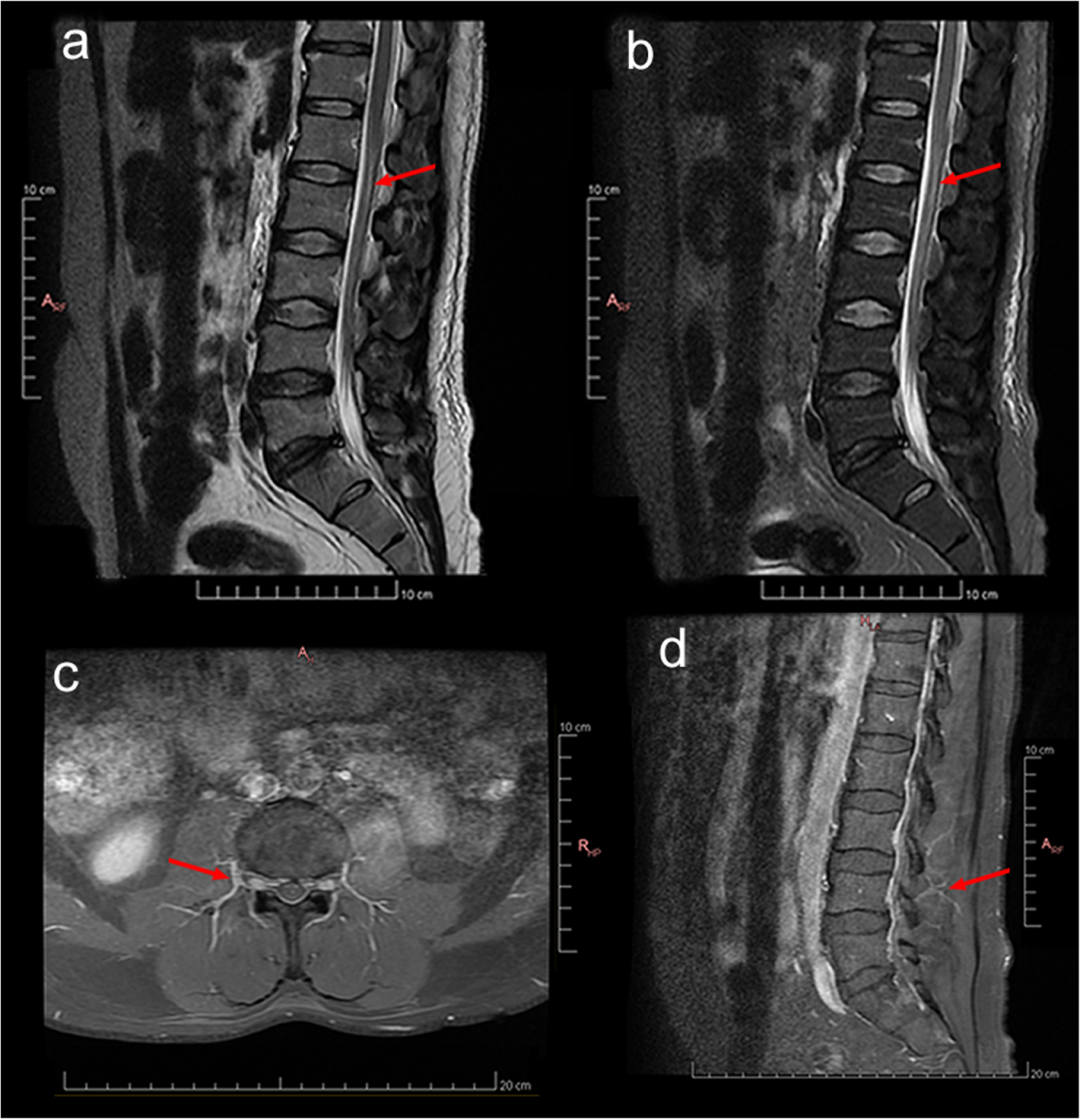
MRI of the lumbosacral column of patient 2. a Sagittal T2 with root hypersignal at the cauda equina level. b Sagittal T2 with fat suppression with hypersignal at the cauda equina level. c Axial T1 with gadolinium contrast with root thickening at the L4 vertebral level and slight gadolinium contrast enhancement. d Sagittal T1 section with root thickening at the L4 vertebral level and slight gadolinium contrast enhancement
